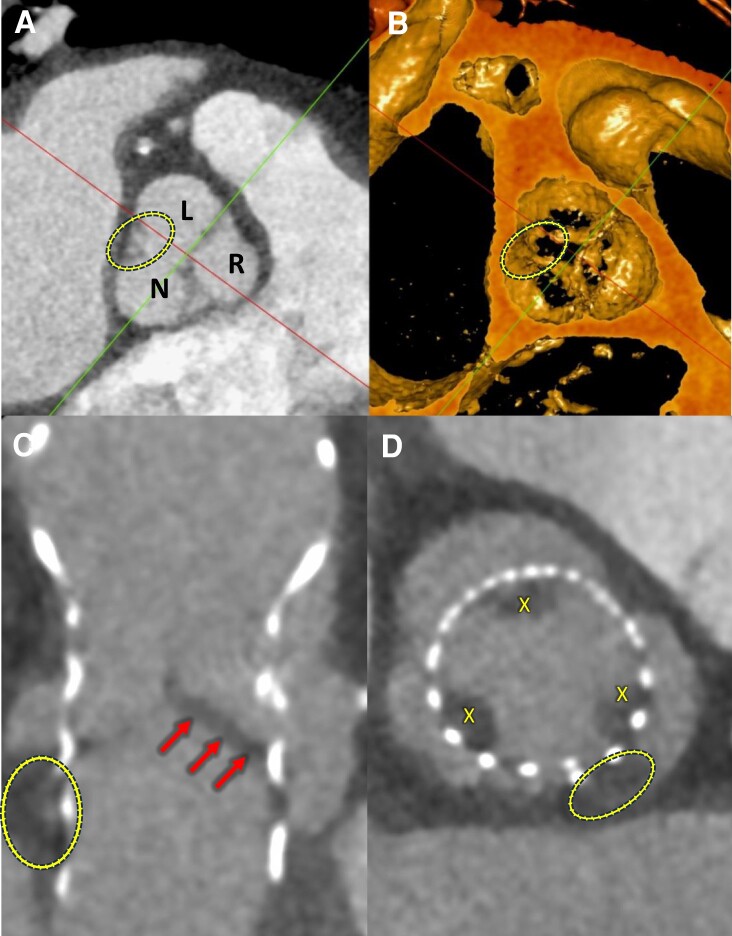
CT reconstructions of the aortic valve. (*A*) Hypoattenuation at the left coronary and non-coronary commissure (circle), suggestive of trauma to the commissure as seen on CT cardiac imaging after angioplasty, and also appreciated on (*B*). (*B*) 3D reconstruction of the aortic valve in cross section. (*C*) Dedicated post-TAVR CT after patient presented with a stroke. This demonstrates the pathognomonic meniscus sign indicating extensive leaflet thickening and hypoattenuating lesions (HALT) at the leaflets (arrows) and the sinuses (circle). (*D*) Extensive HALT of the leaflets (X) and sinuses (circle).
